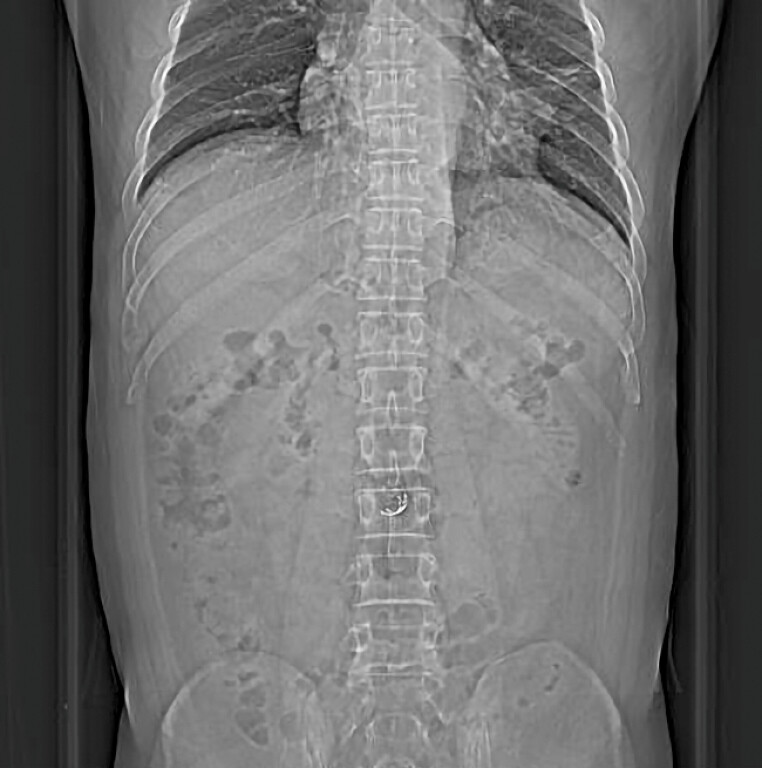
A follow-up abdominal CT scout view showing the absence of free air. CT, computed tomography.
